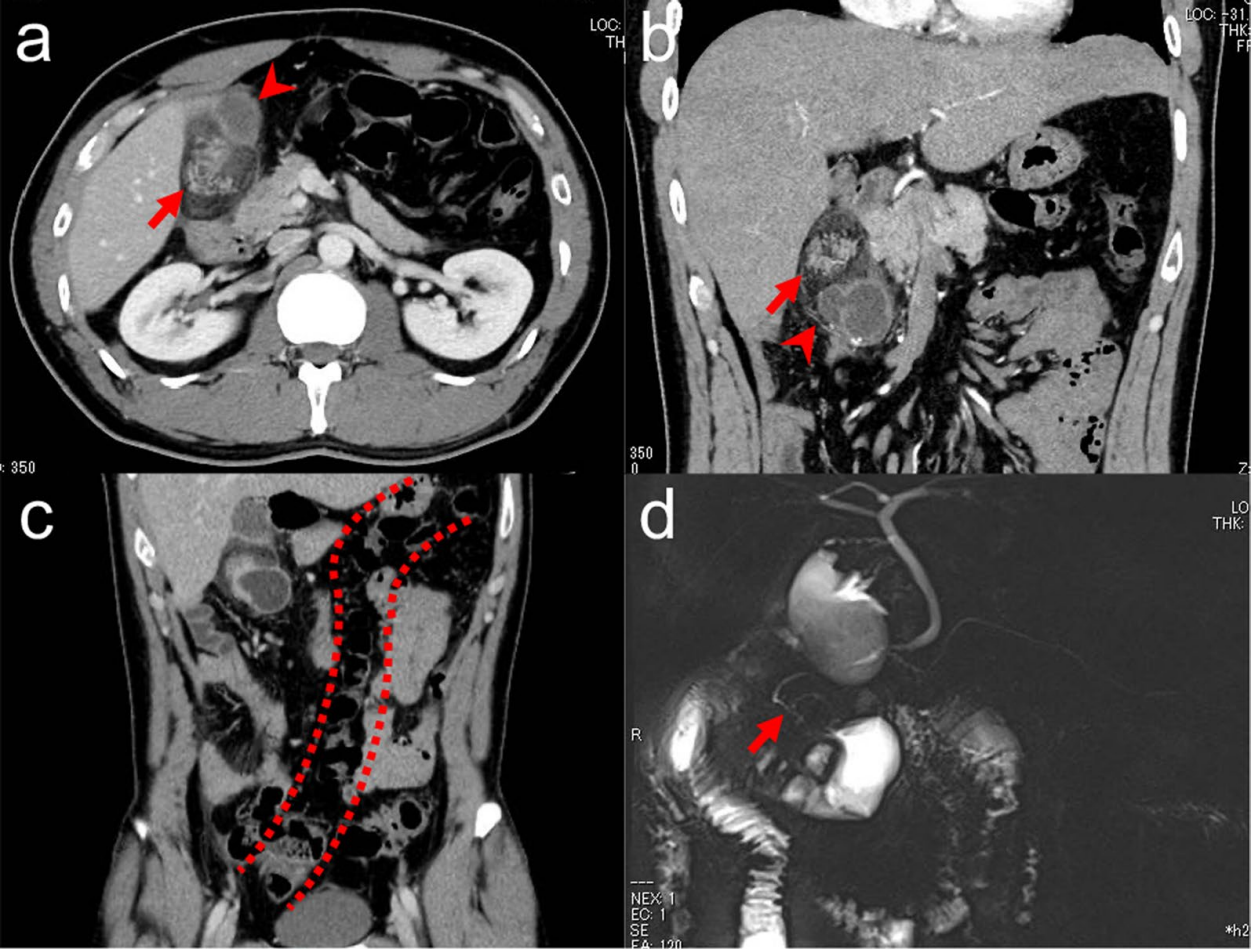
Abdominal contrast-enhanced computed tomography (CT) and magnetic resonance cholangiopancreatography (MRCP) on admission. a The axial CT image shows an oval 30 mm soft tissue mass with microlobulated margins (arrow) below the gallbladder (arrowhead). b The coronal CT shows an oval soft tissue mass with microlobulated margins, suggestive of an ectopic pancreas (arrow) and a cystic lesion with an enhanced thick wall that communicated with the jejunum (arrowhead). c The coronal CT image shows an ascending colon that runs up the midline. d MRCP shows an ectopic pancreatic duct (arrow) without communication to the normal pancreatic duct. The duct drained into the jejunal duplication
